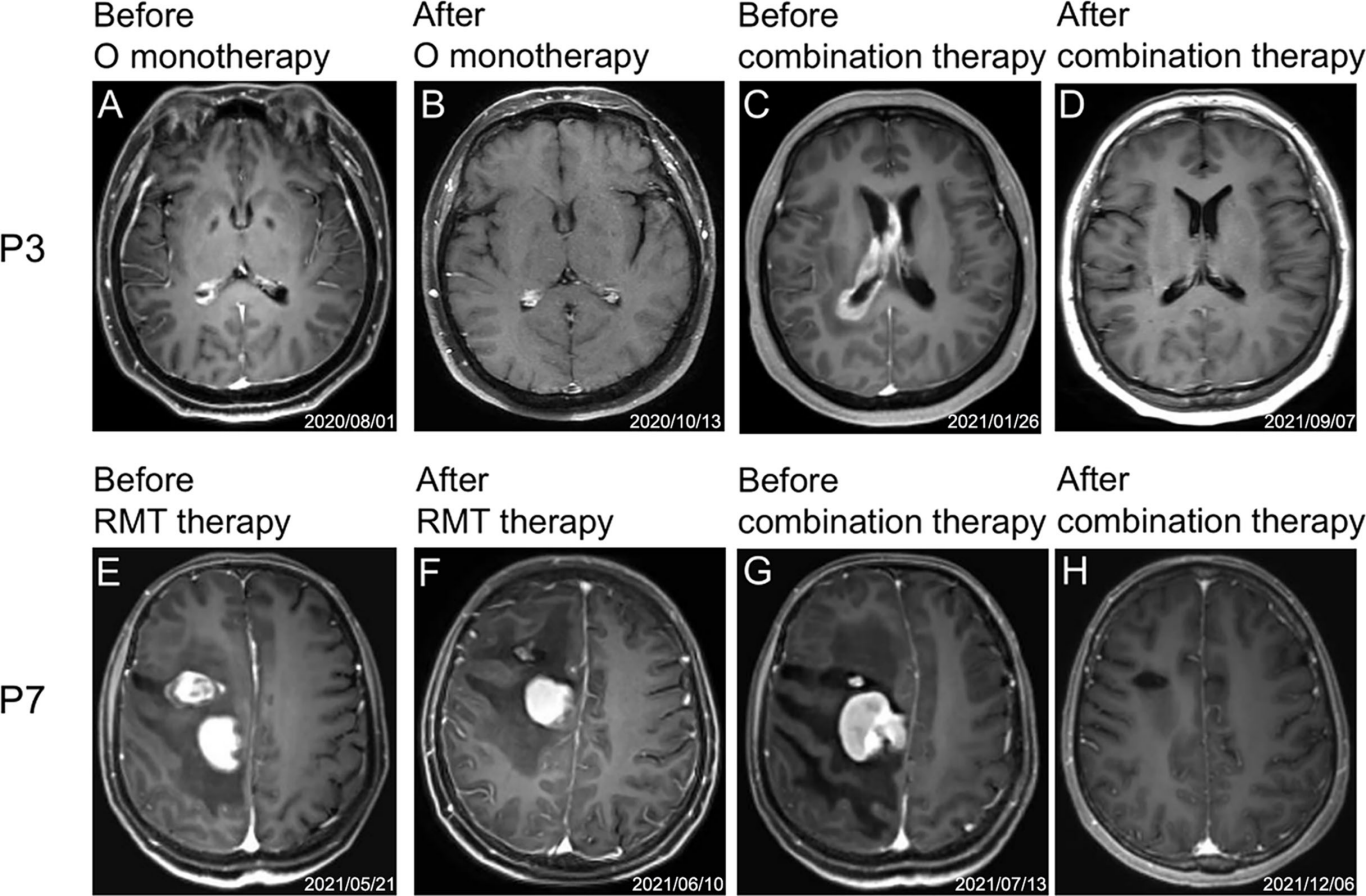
Representative MRI images of two patients (P3 and P7) during treatment. (A–D) and (E–H) are MRI images of the treatment process of P3 and P7, respectively. (A) Before orelabrutinib therapy. (B) After orelabrutinib therapy. (C) Before combination therapy. (D) After combination therapy. (E) Before RMT therapy. (F) After RMT therapy. (G) Before combination therapy. (H) After combination therapy.
